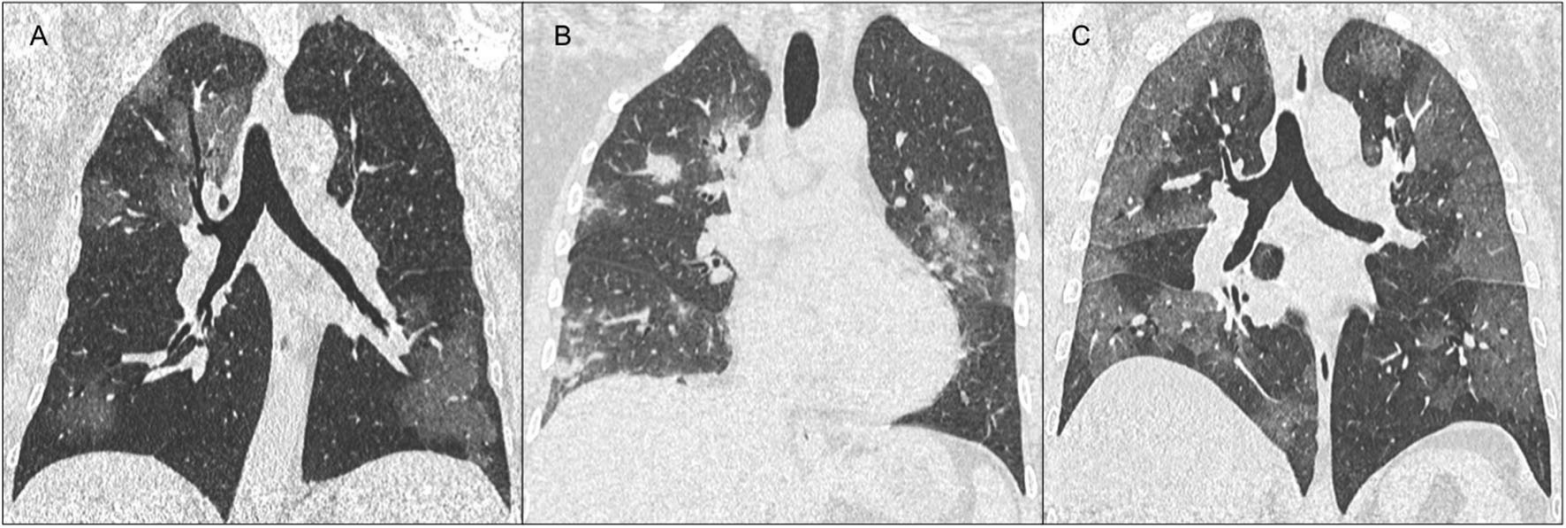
Representative computed tomography (CT) images of various manifestations of the COVID-19. (A) Coronal chest CT images show patchy ground-glass opacities involving both lungs. (B) Ground glass may also appear widespread, confluent, and peripherally distributed. (C) Consolidation and rounded nodules may be also observed in association with ground glass opacities.
